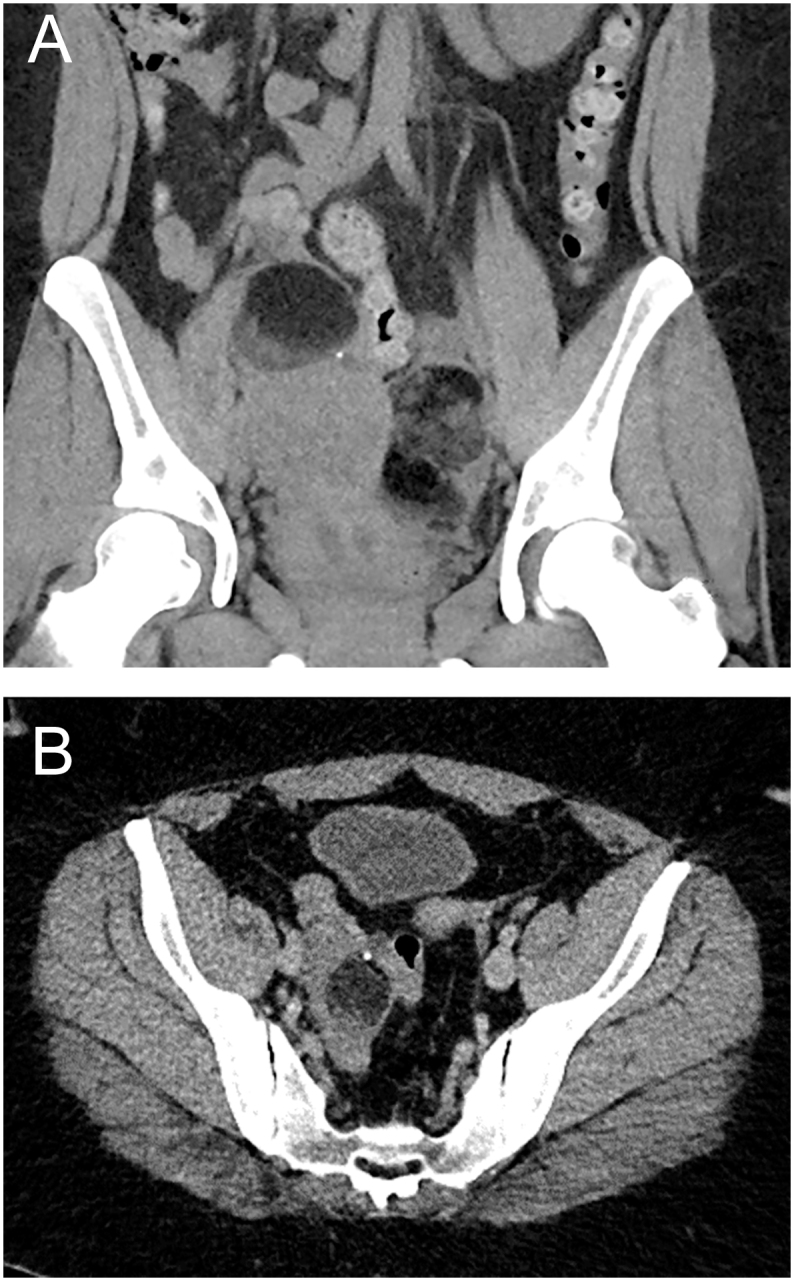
(A) Coronal post-contrast CT abdomen/pelvis showing bilateral ovarian masses containing both soft tissue and fat, in keeping with an ovarian mature cystic teratoma. (B) Left ovarian mass containing soft tissue, fat, and calcification. Soft tissue could represent a Rokitansky nodule within the ovarian MCT.
